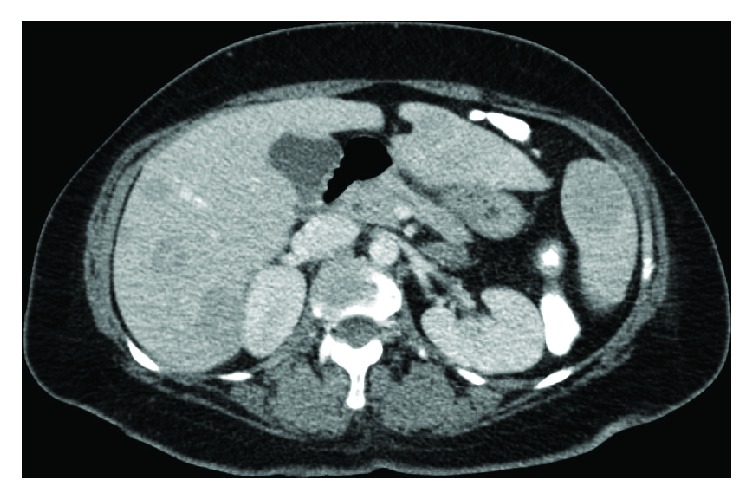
CT of the abdomen showing numerous low attenuation lesions in the liver and spleen suspicious for metastatic disease along with soft tissue nodules in the anterior abdominal wall and numerous lytic lesions in the axial skeleton.
